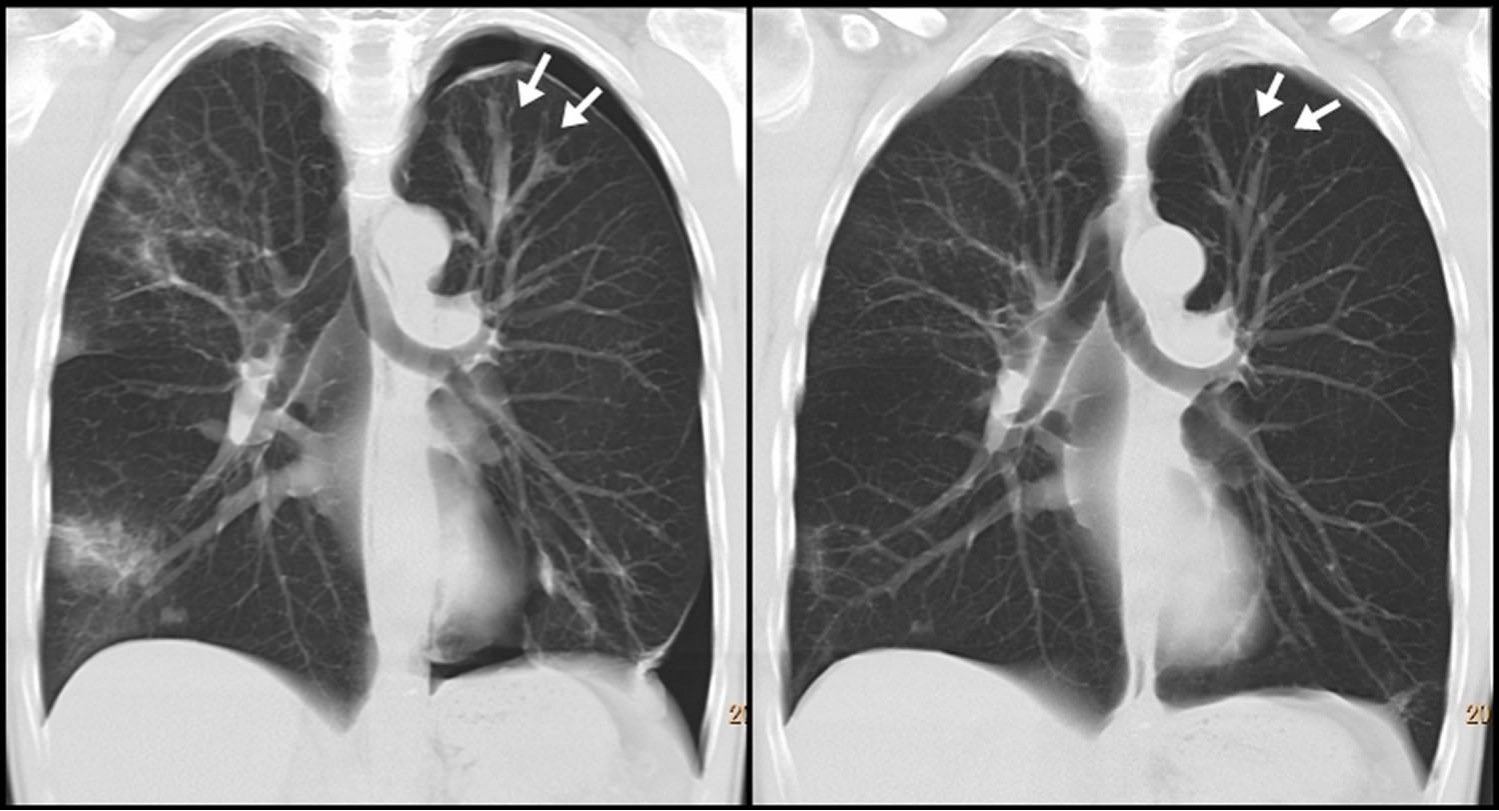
A reformatted coronal CT image demonstrating dilated fluid filled bronchi in the panel on left. The bronchi can be followed centrally. They narrow down and then appear normal. This is well seen in the left upper lobe (arrows). Areas of patchy consolidation are seen bilaterally. A small left pneumothorax is seen which was a complication of the trans-bronchial biopsy. Panel on the right shows a reformatted coronal CT image at the same level six weeks later. The dilated bronchi seen on the earlier scan have markedly improved. This is clearly demonstrated in the left upper lobe (arrows). The areas of consolidation have improved as well. The pneumothorax has now resolved without need for drainage.
